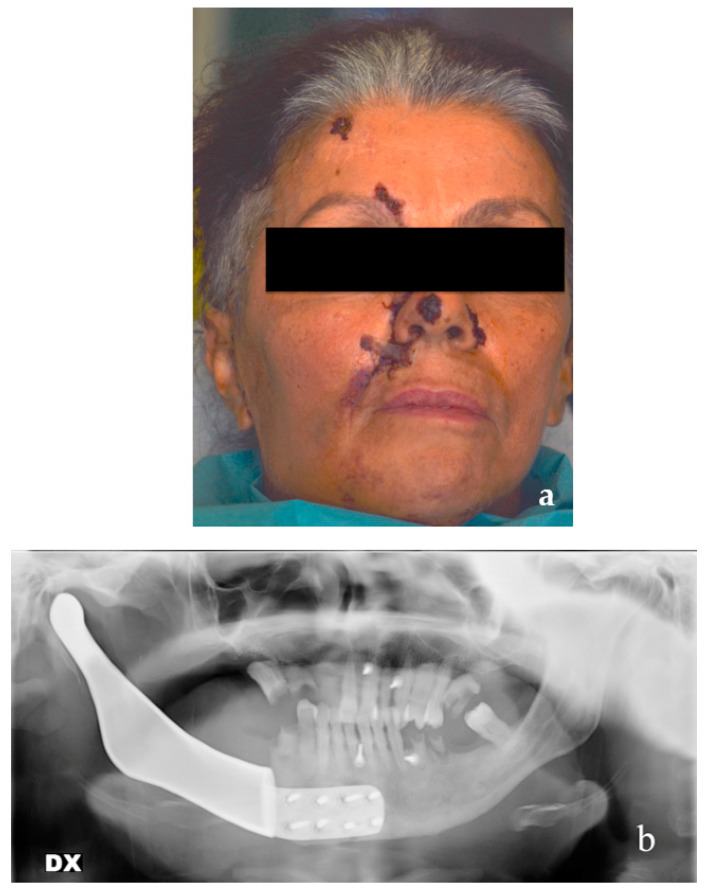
(a) Post-surgical frontal clinical view of the restored symmetry of the lower third of the face. (b) Post-surgery panoramic X-ray of the mandibular prosthesis positioning.
